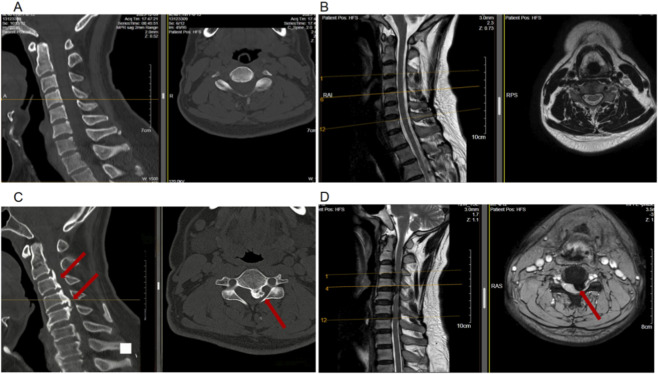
None-Ossification of the posterior longitudinal ligament in Cervical spine CT scan (A), MRI scan (B),and the spinal cord within the spinal canal is not compressed; Ossification of the posterior longitudinal ligament (red arrow) in Cervical spine CT scan (C), MRI scan (D).
